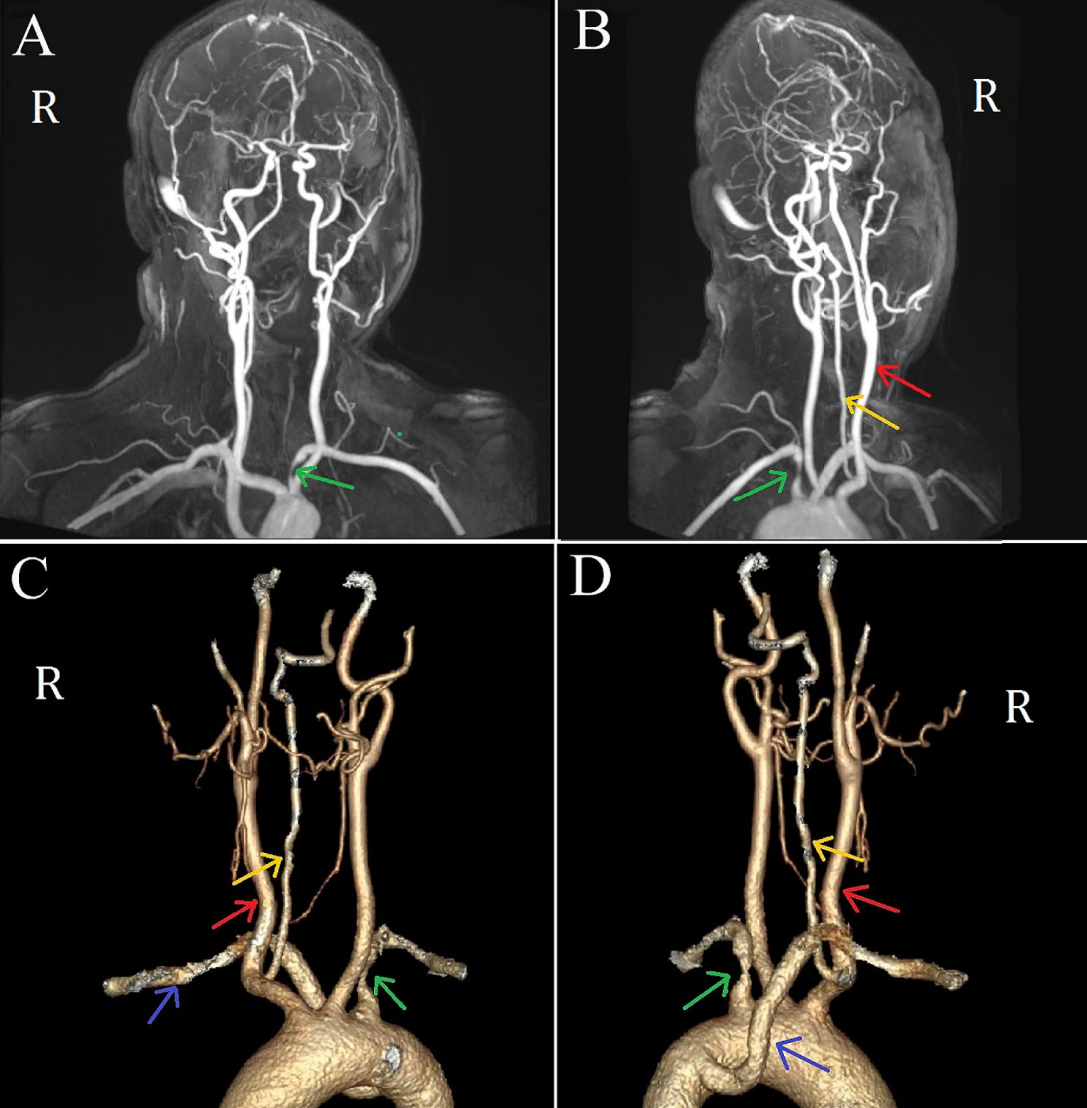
Magnetic resonance angiography (MRA) and computed tomography angiography (CTA) of the head and neck. Imaging reveals narrowing at the origin of the left subclavian artery and the absence of the left vertebral artery (VA). It also shows an aberrant right subclavian artery and the right VA originating from the right common carotid artery (A Anterior view of MRA, B Lateral view of MRA, C Anterior view of CTA, D Lateral view of CTA). Red arrows indicate the right internal jugular vein, yellow arrows indicate the right VA, green arrows indicate the left subclavian artery, and blue arrows indicate the aberrant right subclavian artery
